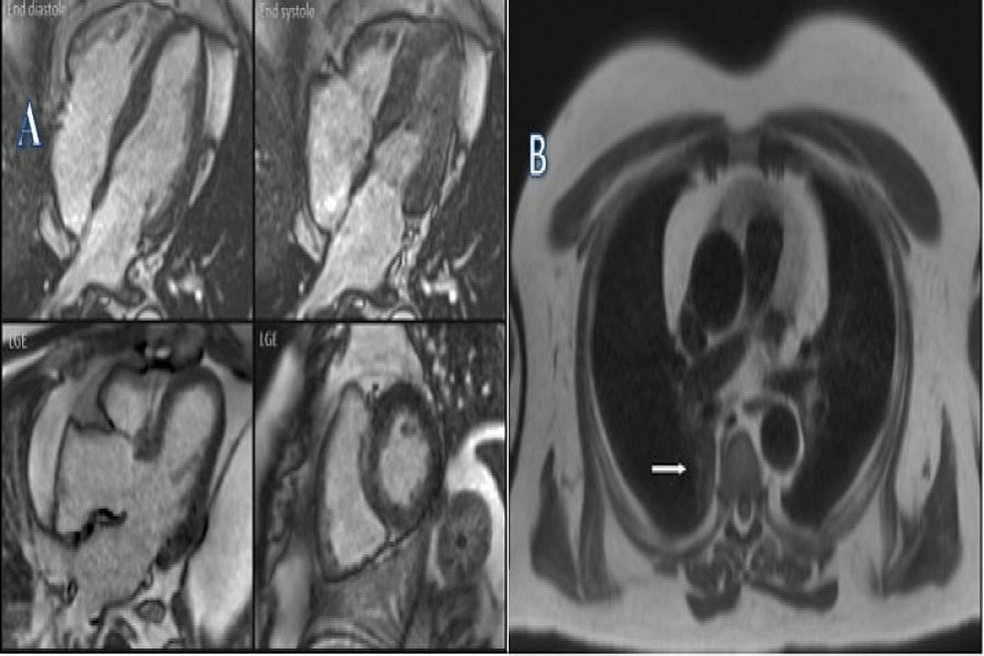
Cardiac MRI A. STIR-T2 images: Mild fibrosis of the basal septum and inferior and lateral walls. No myocardial inflammation or infarction. B. Gadolinium study: In the late phase, there is a mild mid-wall enhancement in the basal septum and inferior and lateral walls. There is an area of increased signal in the posterior right lung (white arrow). STIR: short tau inversion recovery
